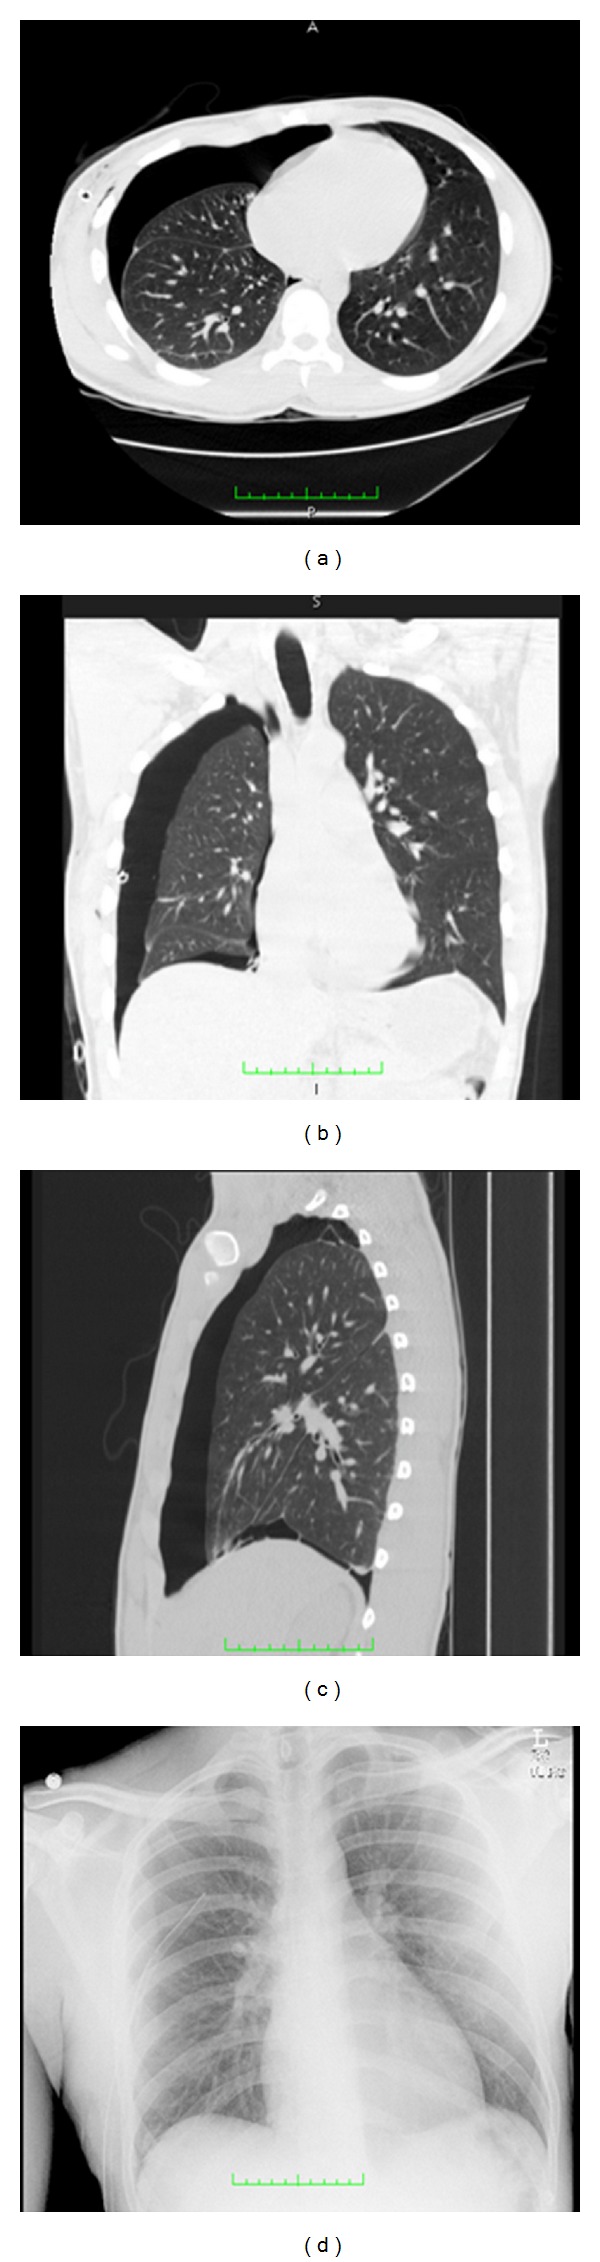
Transverse (a), coronal (b), and sagital (c) CT images of a patient with a moderate to large pneumothorax, and chest X-ray (d) of the same patient taken two hours prior to the time the CT images were acquired. CT images provide significantly better detail for detection and quantification of pneumothorax size.
